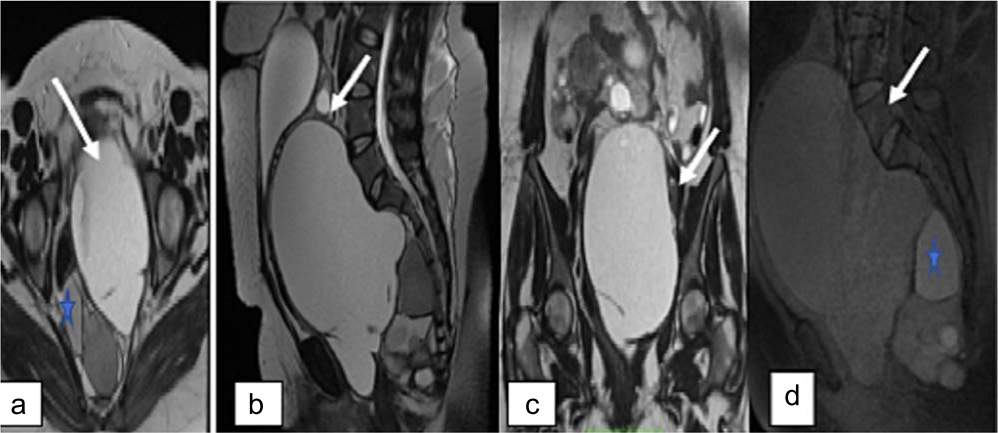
Pelvic MRI. Axial (a), sagittal (b), and coronal (c) T2-weighted images, and sagittal T1-weighted image showing a large multiloculated sacrococcygeal mass containing cystic areas with low T1 signal and high T2 signal (arrow), hemorrhagic components, and fatty components with high T1 and T2 signal (asterisk). No diffusion restriction or contrast enhancement is observed after gadolinium administration.
